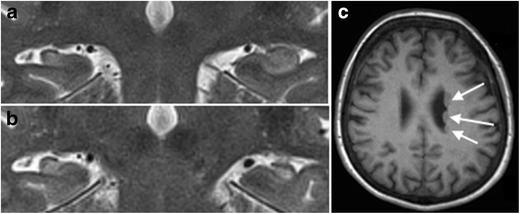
Bilateral hippocampal sclerosis and dual pathology. 3-T coronal T2-weighted image in a 35-year-old patient with medically refractory epilepsy shows volume loss and increased signal intensity of the right hippocampus, compatible with mesial temporal sclerosis (a). The left side the hippocampal volume looks normal, but T2 signal intensity is slightly increased in the CA1 and CA2 sectors and there is blurring of the internal structure (a). On follow-up MRI performed 3 years later, there is clear bilateral hippocampal sclerosis with atrophy and increased T2 signal intensity of both hippocampi (b). Axially reconstructed 3D MPRAGE images (c) show multiple periventricular heterotopias (*white arrows*) along the lateral wall of the left lateral ventricle
